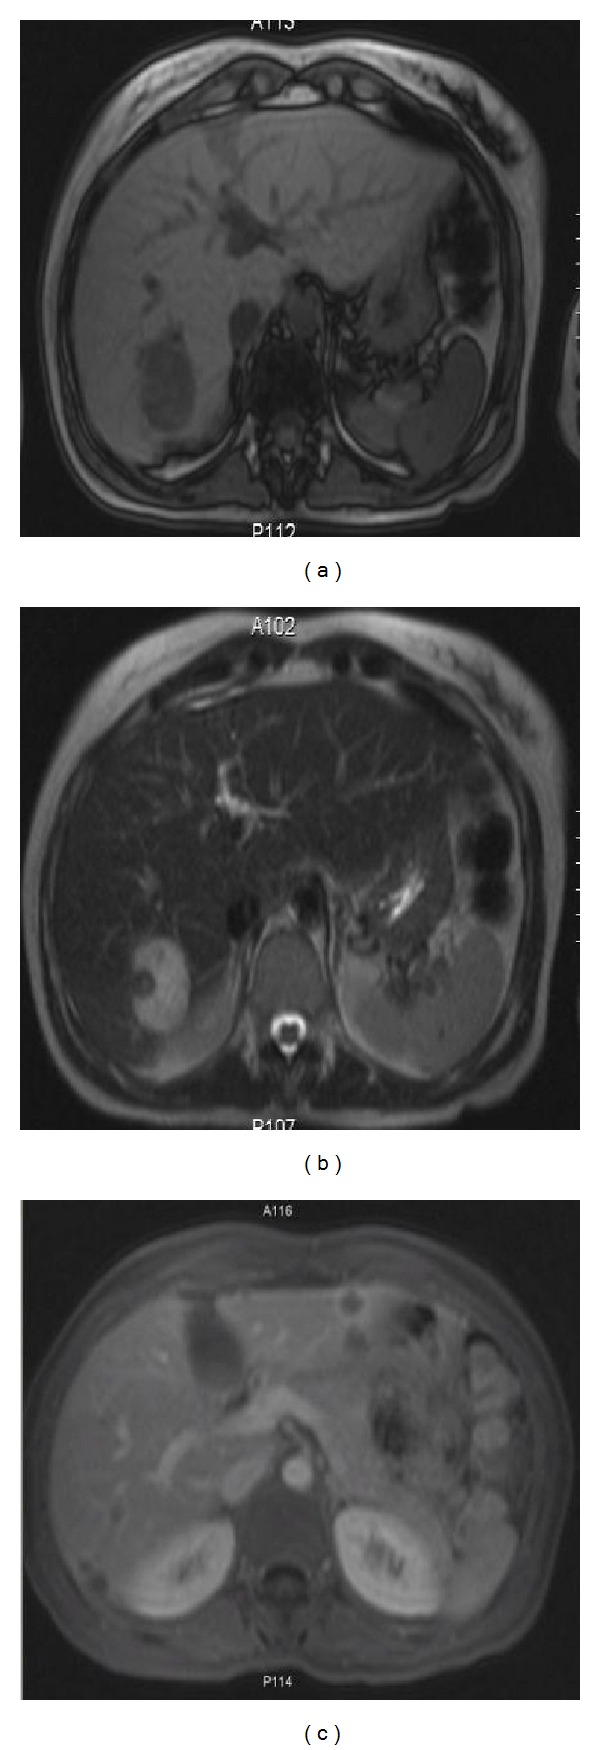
(a) T1-weighted MRI abdomen images showing hypointense lesions. (b) T2-weighted images showing a hyperintense lesion in the right liver lobe. (c) Arrow shows peripheral ring enhancement after intravenous administration of gadolinium.
